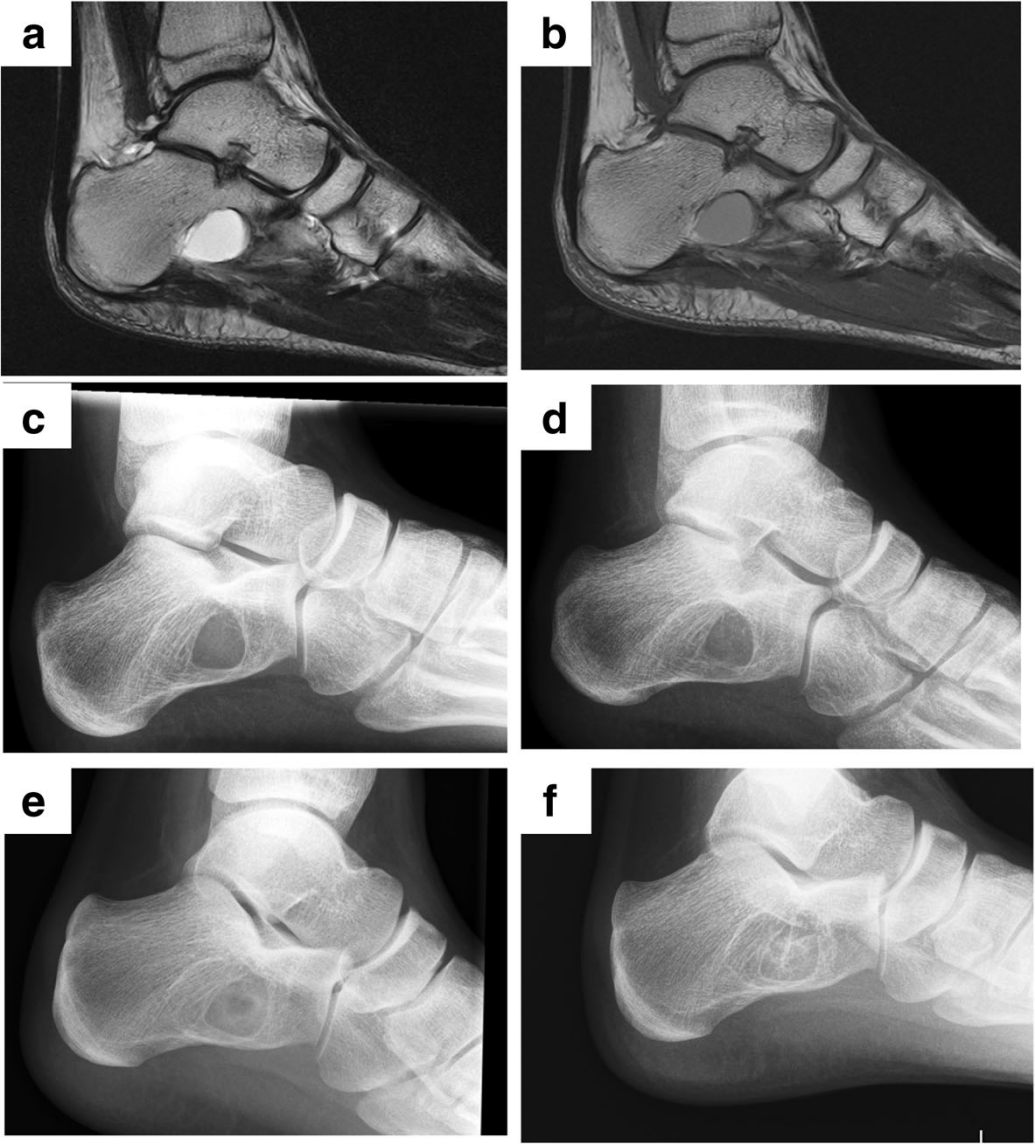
Typical case of simple bone cyst in the calcaneus treated with endoscopic curettage. An 8-year-old boy experienced heel pain without an apparent cause (a T1-weighted MRI; b T2-weighted MRI; c X-ray). Endoscopic curettage C was performed via two portals (d postoperative image). After 3 months, healing was confirmed with consolidation of the cyst (e), and the cavity was completely filled with new bone 6 years after the operation (f, class A)
